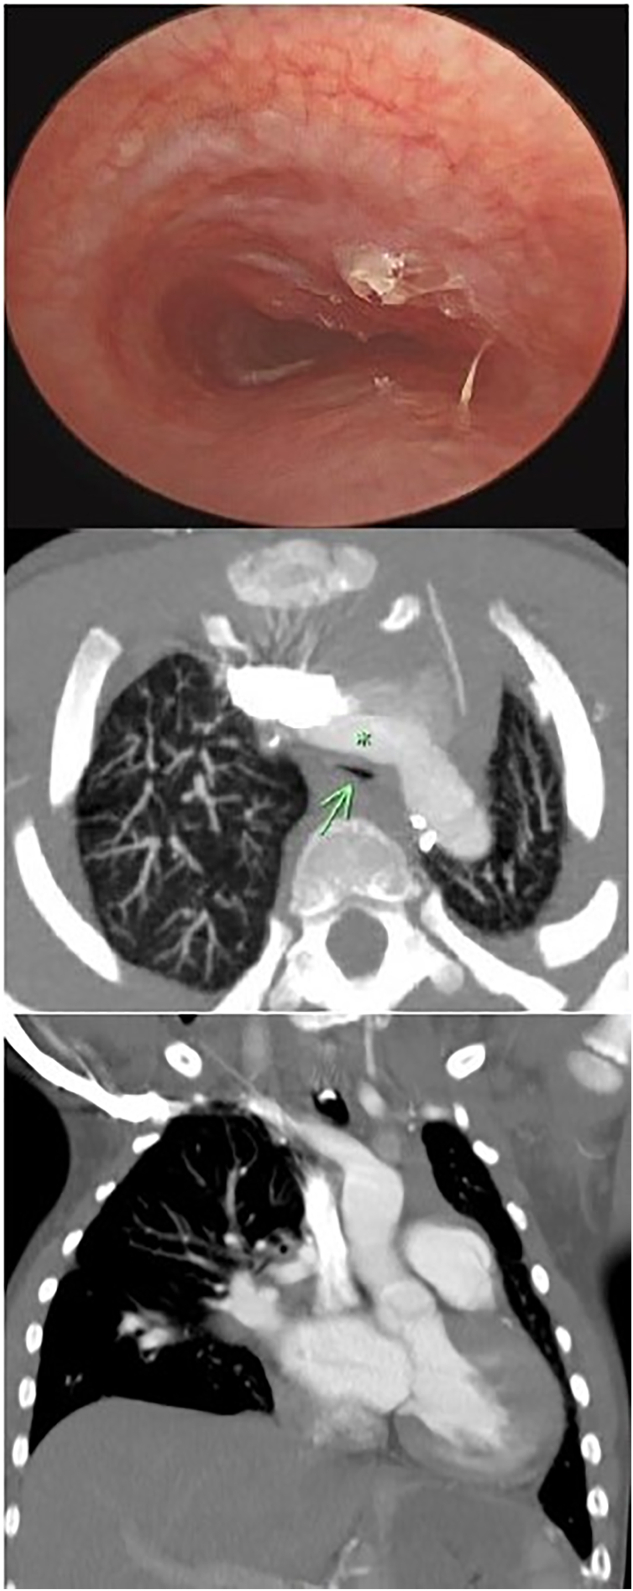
Preoperative bronchoscopy and computed tomography angiography demonstrating severe compression of trachea (arrow) by innominate artery (asterisk).
